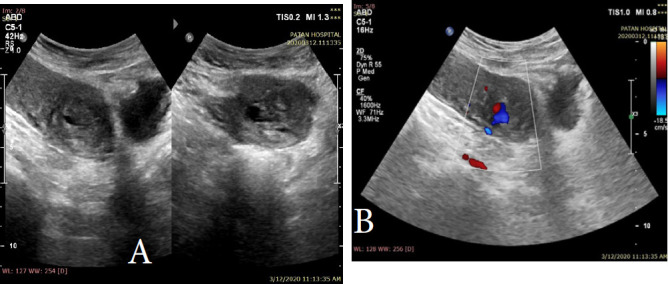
(A) 2D ultrasonography shows heterogeneous hypoechoic area in the lower uterine segment suggestive of hematoma. (B) Color Doppler shows turbulent flow (ying-yang sign) indicating pseudoaneurysm.
